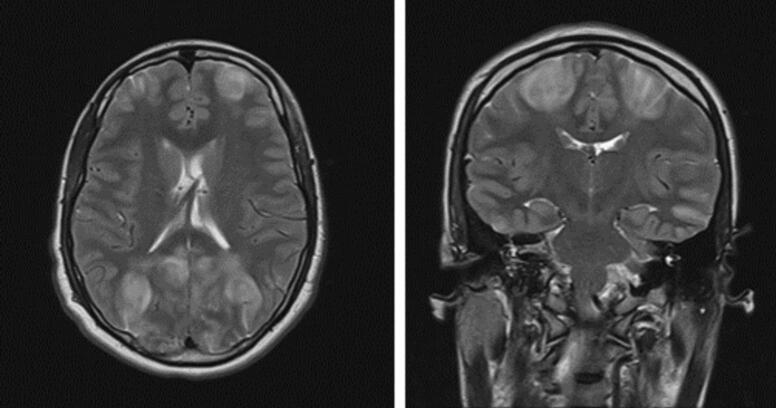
MRI Brain T2 weighted imaging (axial and sagittal views) demonstrating hyperintensities, in keeping with cerebral edema, involving the frontal and occipital lobes bilaterally.
